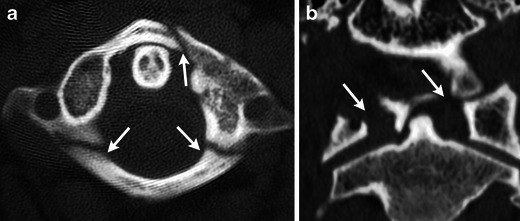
Jefferson fracture. (a) Axial CT through C1 after a motor vehicle collision demonstrates a burst fracture involving the left anterior arch and bilateral posterior arches of C1 at their junctions with the lateral masses (white arrows). (b) Coronal MDCT image in another patient shows widening of the distance between the dens and the lateral masses of C1 (white arrows)
